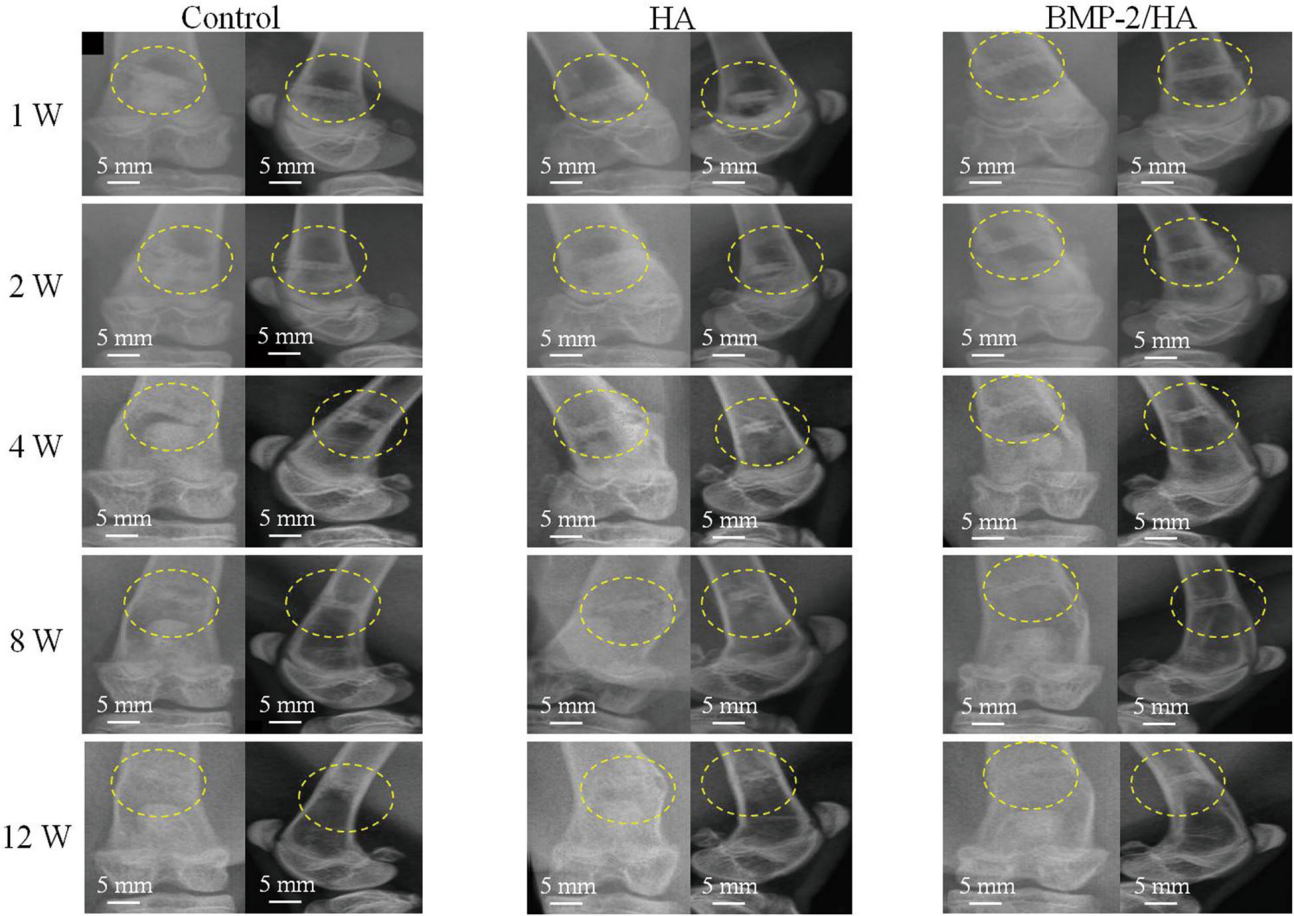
Anteroposterior and lateral view radiographic images of the experimental region. Images were taken from 1 to 12 weeks postoperative. The yellow circles indicate the position of the screw
